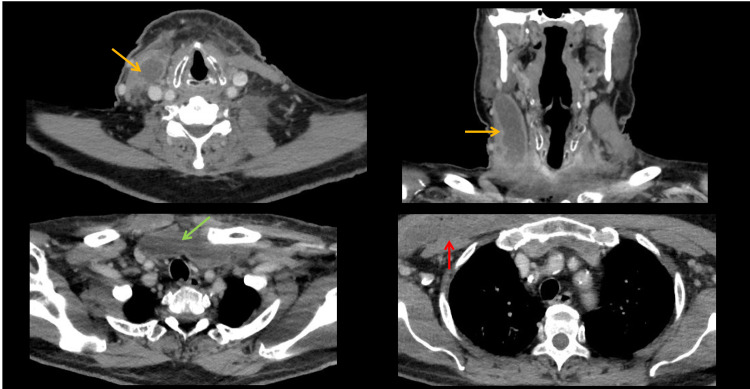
CT neck and chest imaging Orange arrow points to the right sternocleidomastoid abscess. Green arrow points to anterior mediastinal fluid collection. Red arrow points to right pectoralis major abscess.
